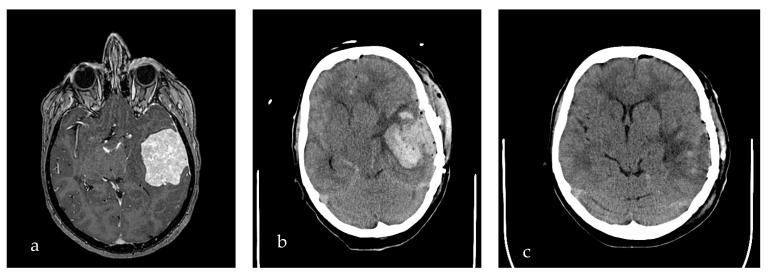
Sample postoperative hemorrhage in the resection cavity, necessitating revision surgery in the early postoperative period. (a) Contrast T1-WI preoperative image of left temporal meningioma. (b) CT-image depicting intraparenchymal hemorrhage in the resection cavity. (c) Follow-up CT-image two days after surgical hematoma evacuation.
